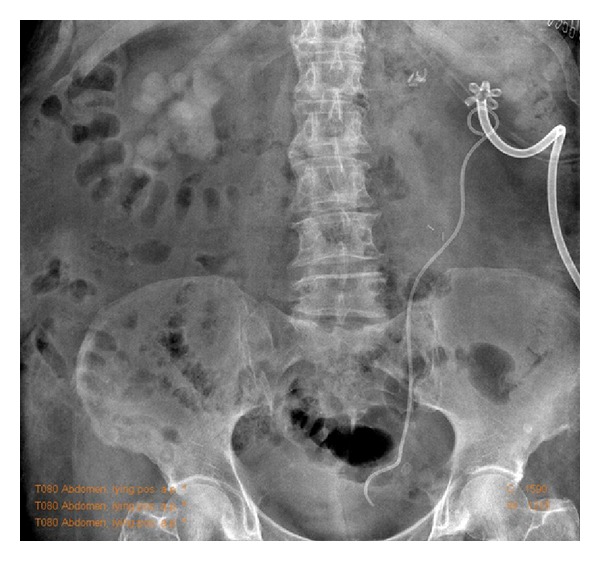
Postoperative abdominal X-ray.
